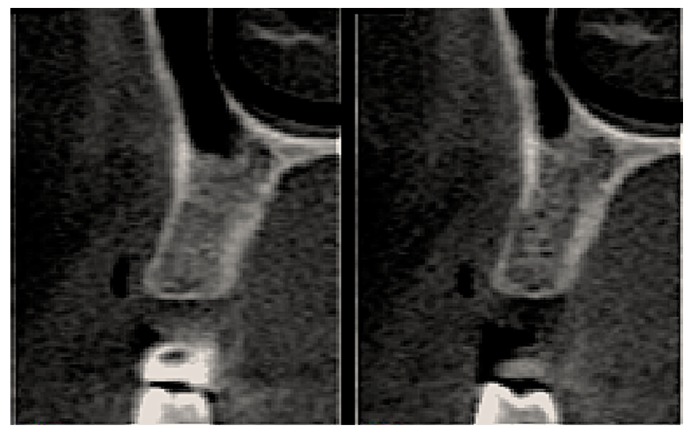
CT images of tooth 14 (TG1) 4 months after the procedure.
